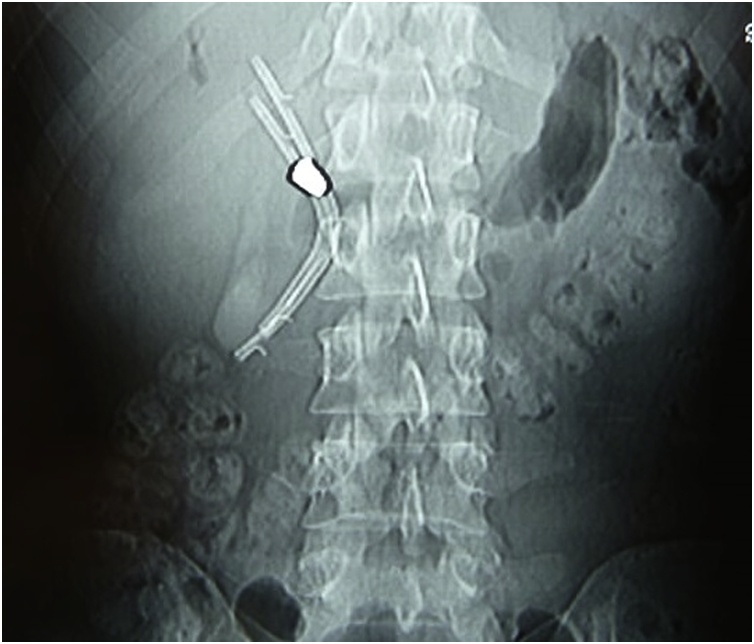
Control radiography after ERCP with the biliary duct stent.
